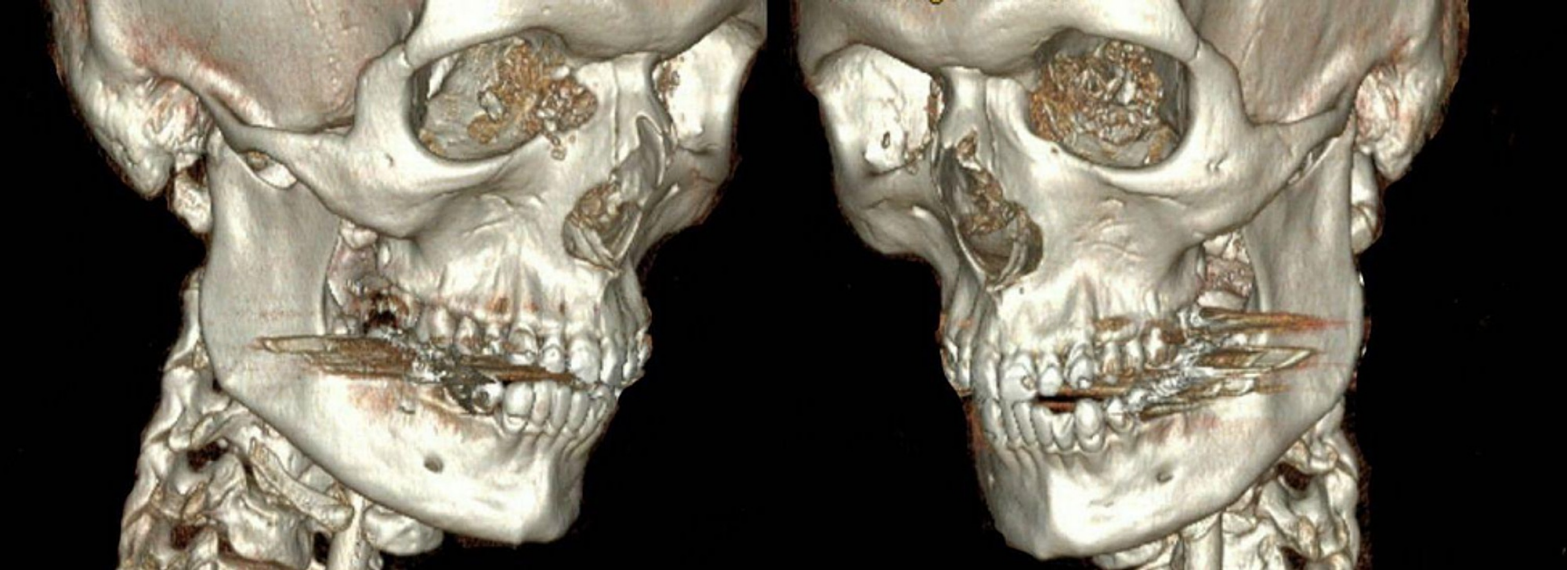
Three-dimensional reconstruction of patient’s admission computed tomography scan demonstrating severe comminution of medial orbital walls bilaterally
